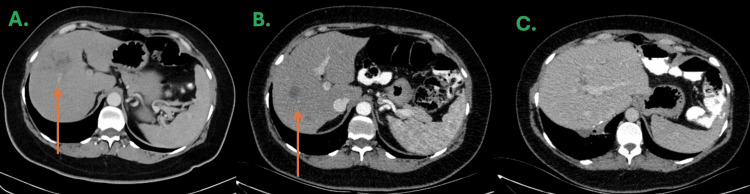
CT scans at baseline (A), after 10 cycles of pembrolizumab (B), and one year post–right hepatectomy (C), showing sustained absence of disease recurrence.
